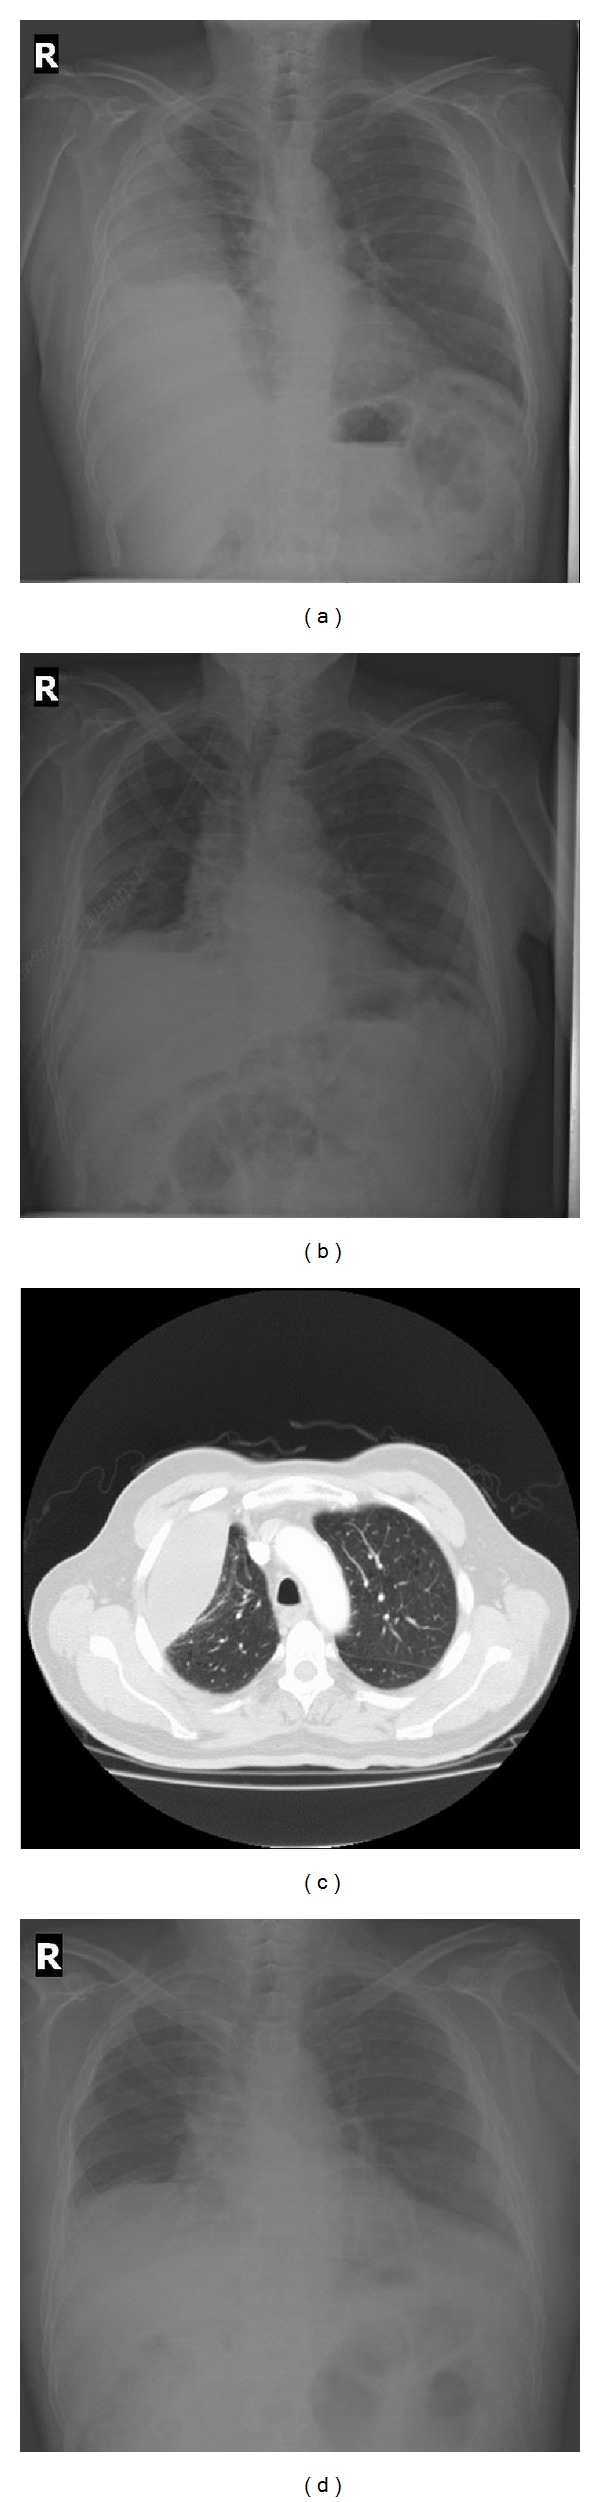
Posteroanterior chest X-ray in admission (a), after catheter thoracostomy (b) and thorax tomography (c), and posteroanterior chest X-ray after steroid treatment (d).
